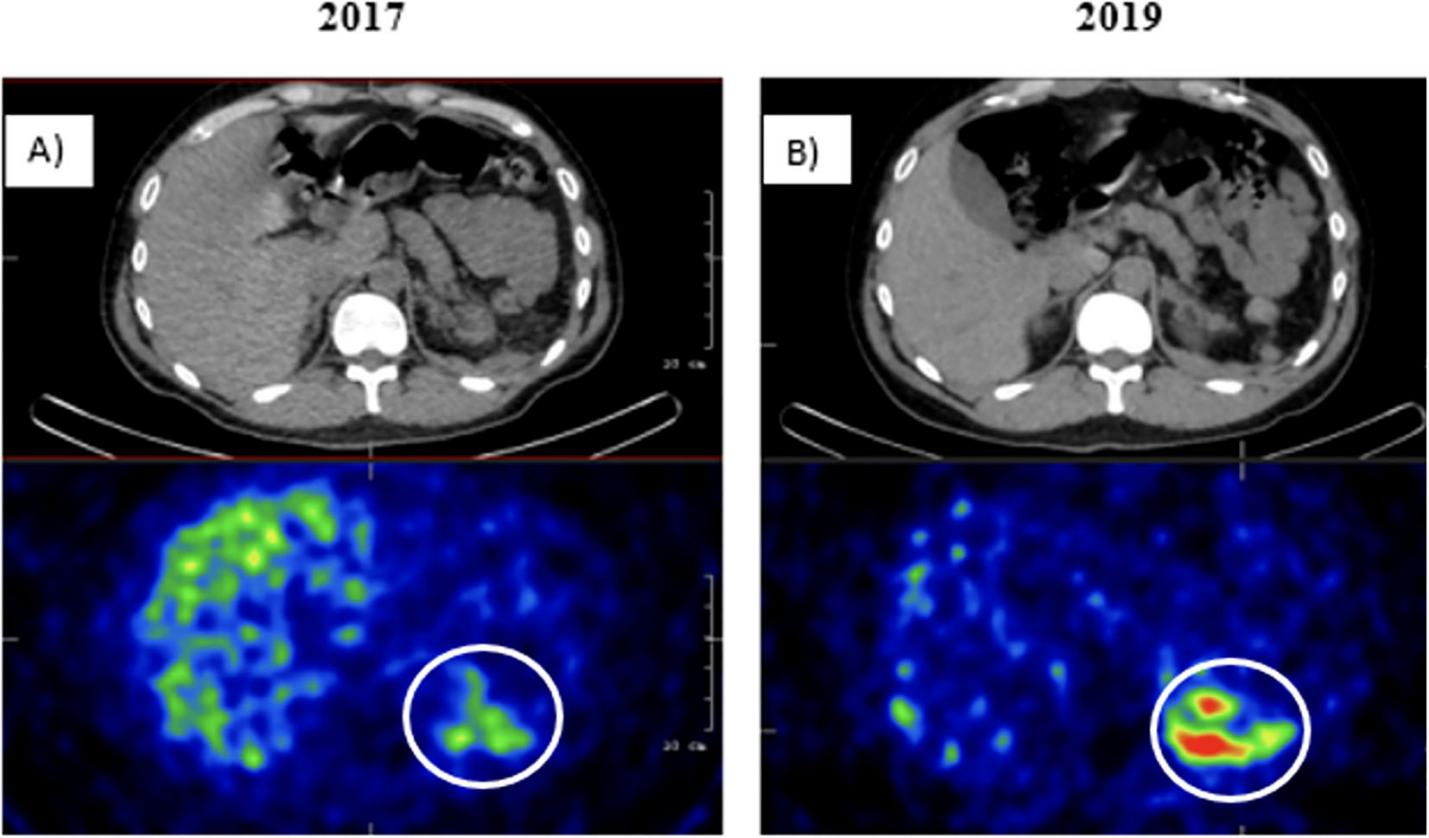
([^131^I]MIBG) scintigraphy SPECT/CT images in (A) 2017 and (B) 2019 show multiple peritoneal lesions with significant radiopharmaceutical uptake in the left hypochondrium. No distant metastases were detected.
